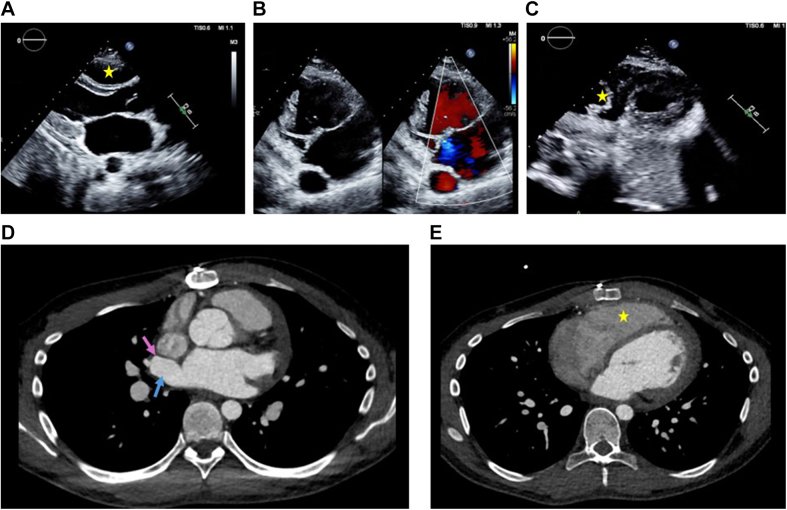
Postoperative Evaluation of Repaired Sinus Venosus Atrial Septal Defect and Partial Anomalous Pulmonary Venous Return (A) Parasternal long-axis view on transthoracic echocardiography showing decreased right ventricle size (yellow star); (B) right ventricle inflow view showing decreased tricuspid regurgitation; (C) short-axis view on transthoracic echocardiography showing decrease in right ventricle size (yellow star); (D) cardiac computed tomography patent right-sided pulmonary venous baffle (blue arrow) with closed superior sinus venosus defect (purple arrow); (E) cardiac computed tomography showing decrease in right ventricle size (yellow star).
